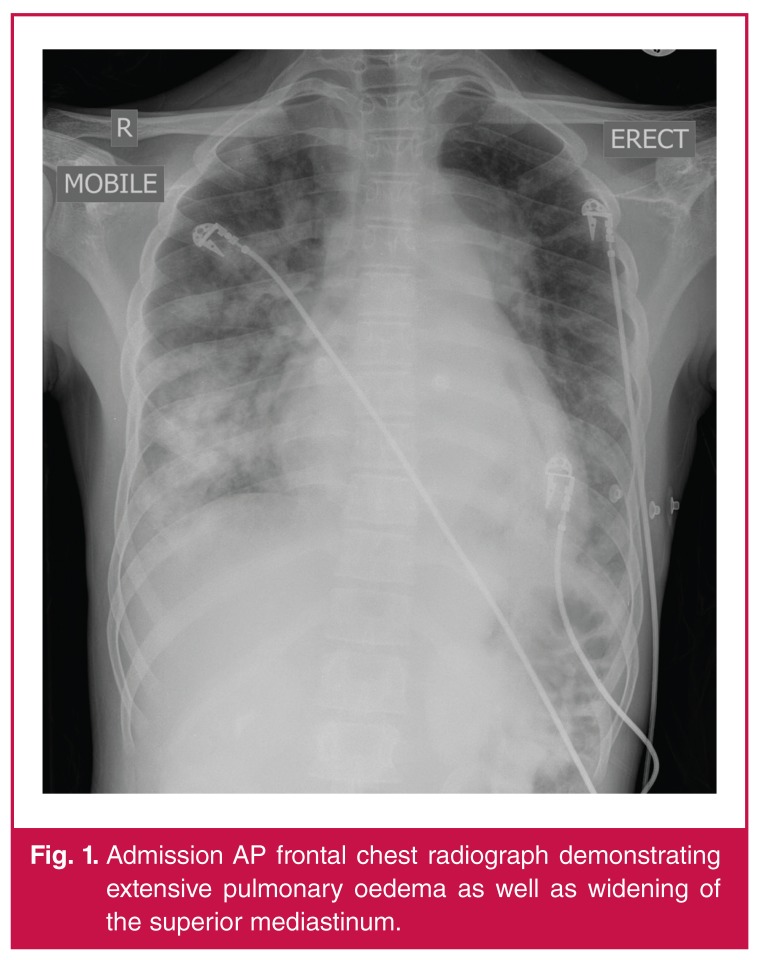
Admission AP frontal chest radiograph demonstrating extensive pulmonary oedema as well as widening of the superior mediastinum.
